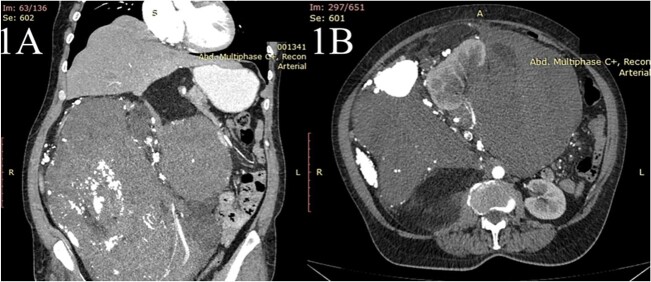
The contrast-enhanced computed tomography (CT) scan shows (A) a huge mass extending from the sub-hepatic region to the pelvis with the cranial displacement of the liver and (B) the same mass causing encasement the right kidney.
